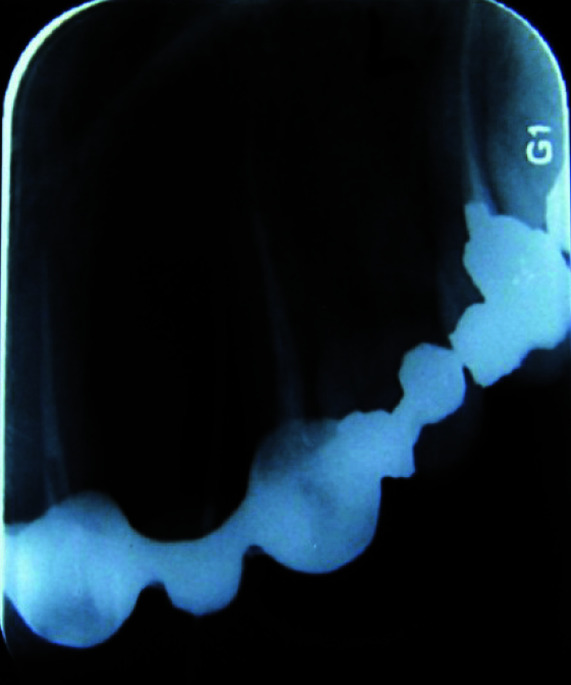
The first radiographic view of the lesion in the early stage.
